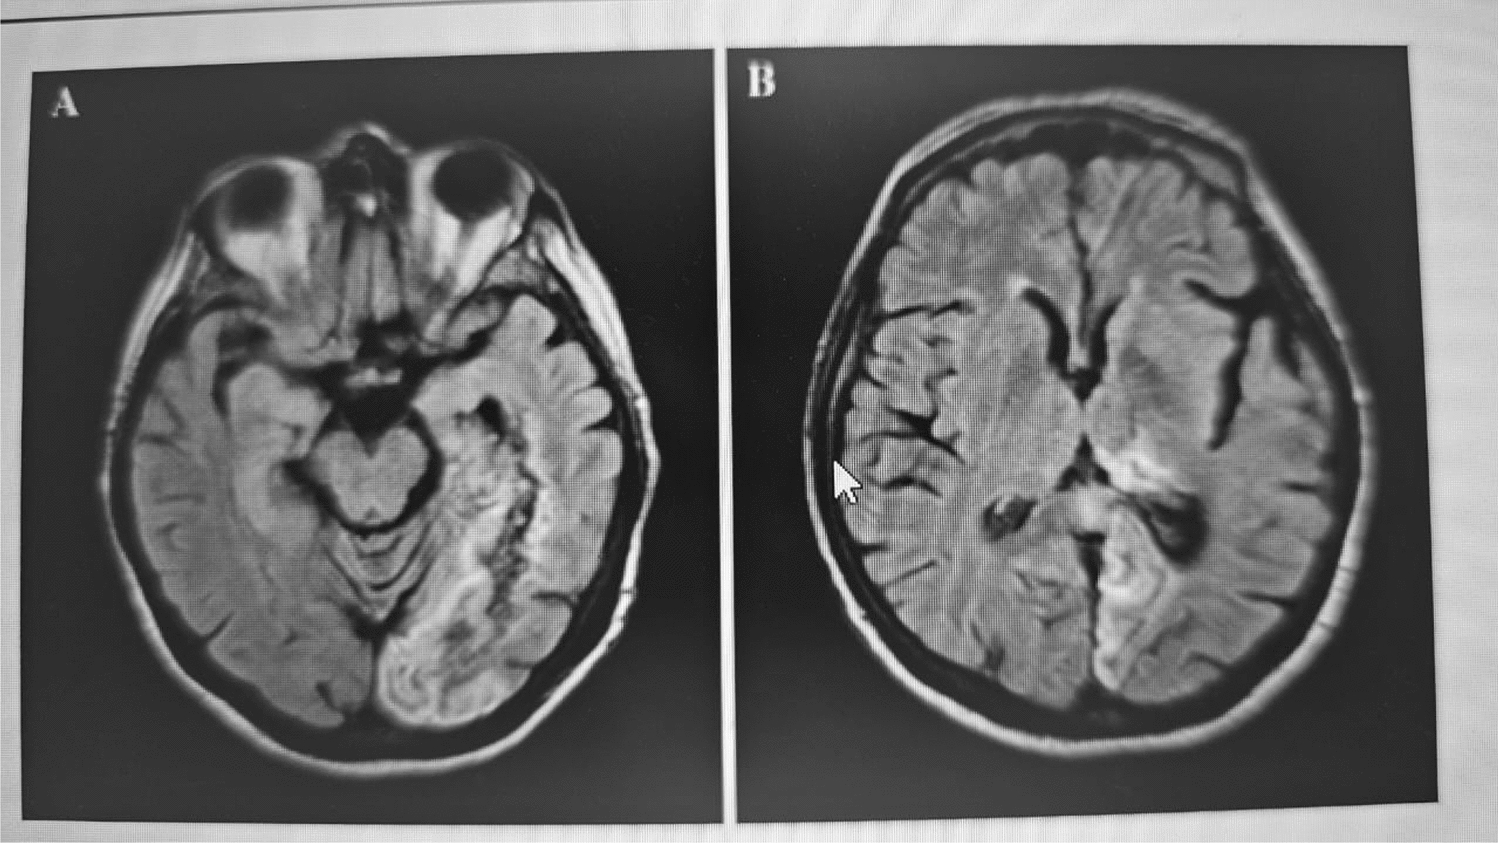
Follow-up FLAIR MRI of left posterior cerebral artery (PCA) territory: gliotic changes in temporo-occipital region (A) & posterior thalamus (B); FLAIR: Fluid Attenuated Inversion Recovery [46]
